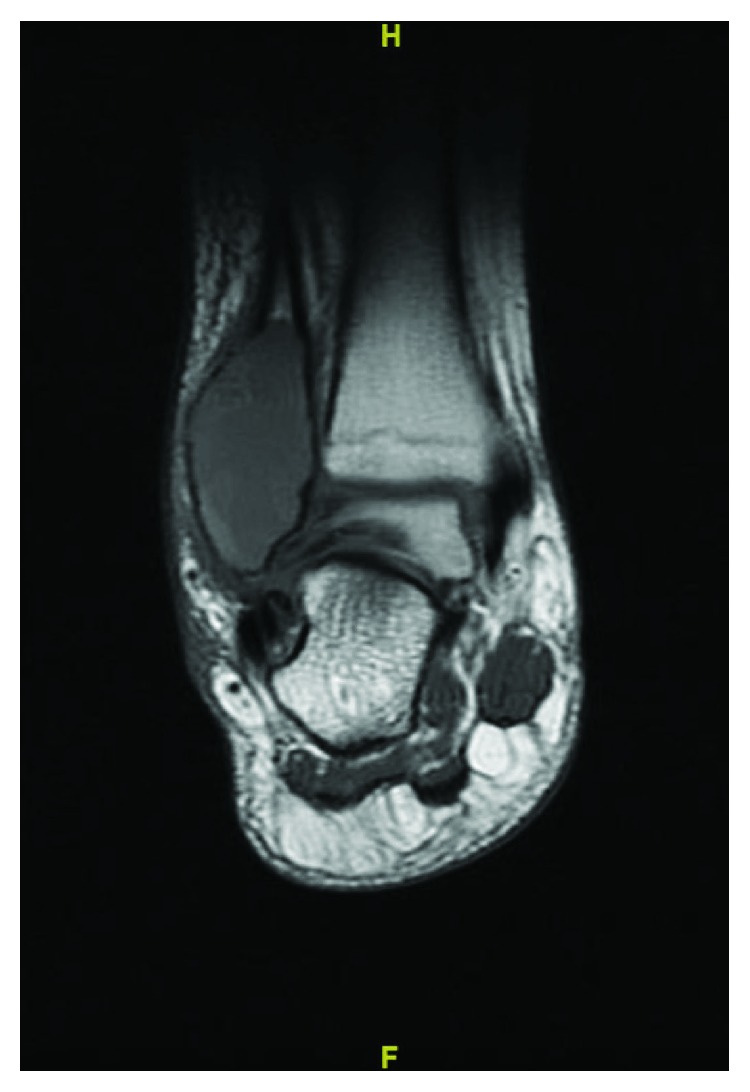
T1 weighted MRI of the ankle showing 5 × 5.2 × 3.6 cm multiloculated, slightly hyperintense compared to surrounding muscle expansile lesion.
